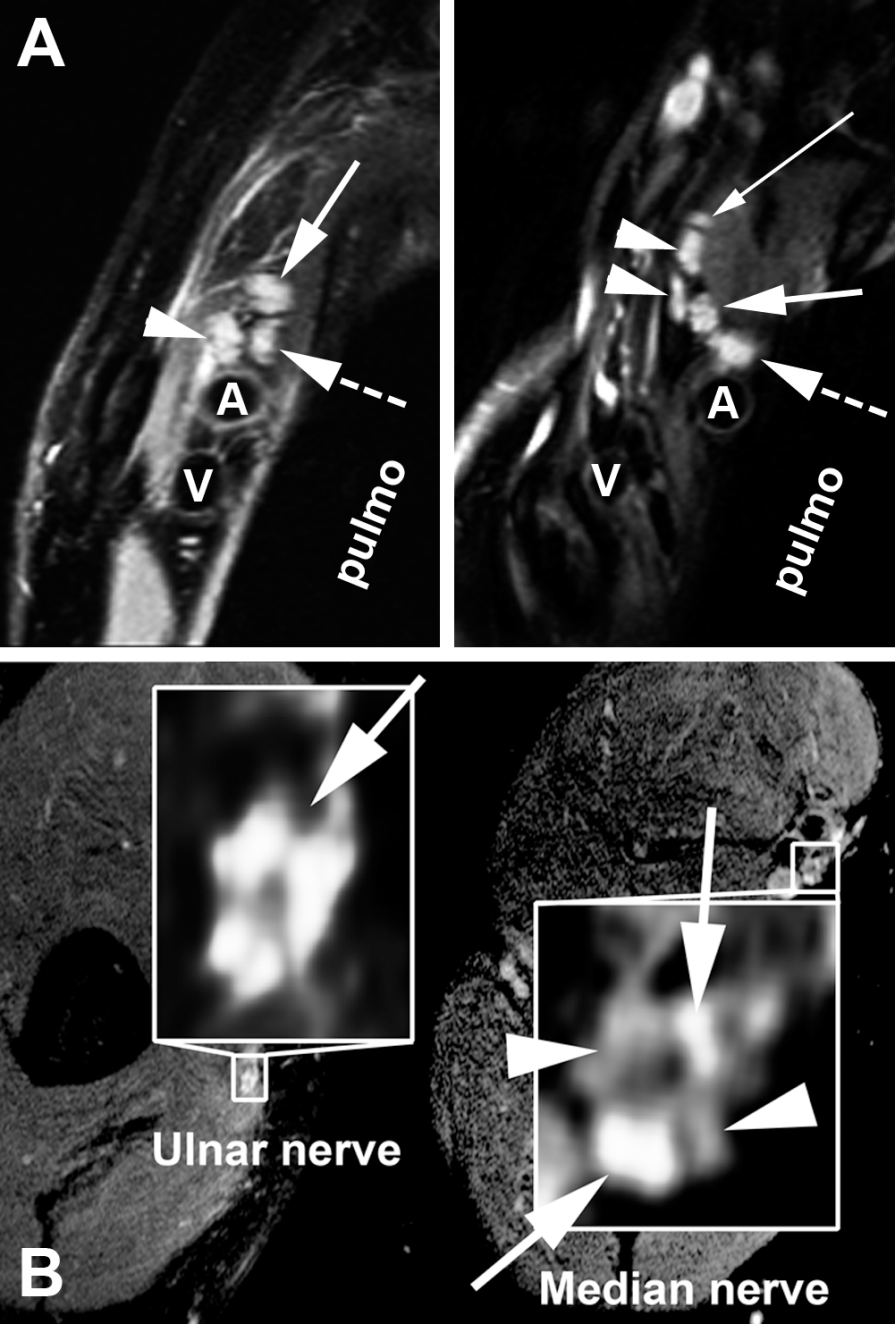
High-resolution magnetic resonance neurography in meningoencephaloradiculitis. HrMRN in plexus localisation (A): Pathological signal hyperintensities in high-resolution, T2-weighted coronal images of the brachial plexus. Left: The same patient as in Fig 2E and 2F. A representative section with perpendicular orientation to the lateral cord (arrowhead), medial cord (dashed arrow) and posterior cord is shown. The cords display strongly increased T2 signal and are swollen, indicative of a clinically relevant injury (high-grade flaccid tetraparesis, areflexia, no sensory deficit). Right: A 69-year-old female patient with high-grade flaccid paresis of the left arm. A representative section through the supraclavicular plexus at trunk level is shown, with strong T2 signal increase and calibre gain. The thin solid white arrow indicates the dorsal scapular nerve. The two arrowheads show the anterior and posterior divisions of the superior trunk; the thick solid white arrow, the middle trunk; the thick dashed white arrow, the inferior trunk. In both images, the letters A and V denote the subclavian artery and vein, respectively. "Pulmo" denotes the lung apex. hrMRN for peripheral nerve lesion localisation (B): Pathological signal hyperintensities in high-resolution, T2-weighted, axial images at upper arm level in a 62-year-old male patient suffering from flaccid paresis of the right arm. Left: A representative magnified section of the ulnar nerve, with a strong T2 lesion of the ulnar nerve fascicles (solid white arrow on left). Right: A zoomed, representative section of the median nerve of the same patient. The median nerve, in contrast to the ulnar nerve, shows a T2 lesion of some, but not all fascicles of its cross section. The arrowheads on the right indicate fascicles with normal/hypointense T2 signal, whereas the solid white arrows on the right designate lesion fascicles with clearly increased T2 signal. This imaging finding is in close agreement with the clinical symptoms of predominant ulnar neuropathy. Spatial resolution was 0.3–0.6 × 0.3–0.6mm in-plane at a slice thickness of 3–3.5mm.
